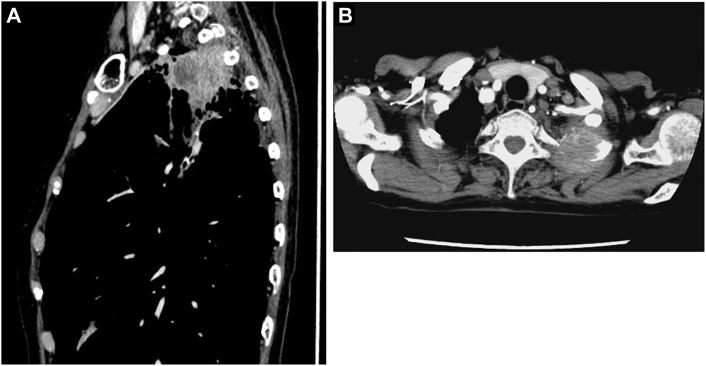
(A) Sagittal computed tomography image depicting a necrotic tumor extending to the apex of the thoracic cavity. (B) Contrast-enhanced computed tomography image showing a 56-mm tumor invading the second rib.
